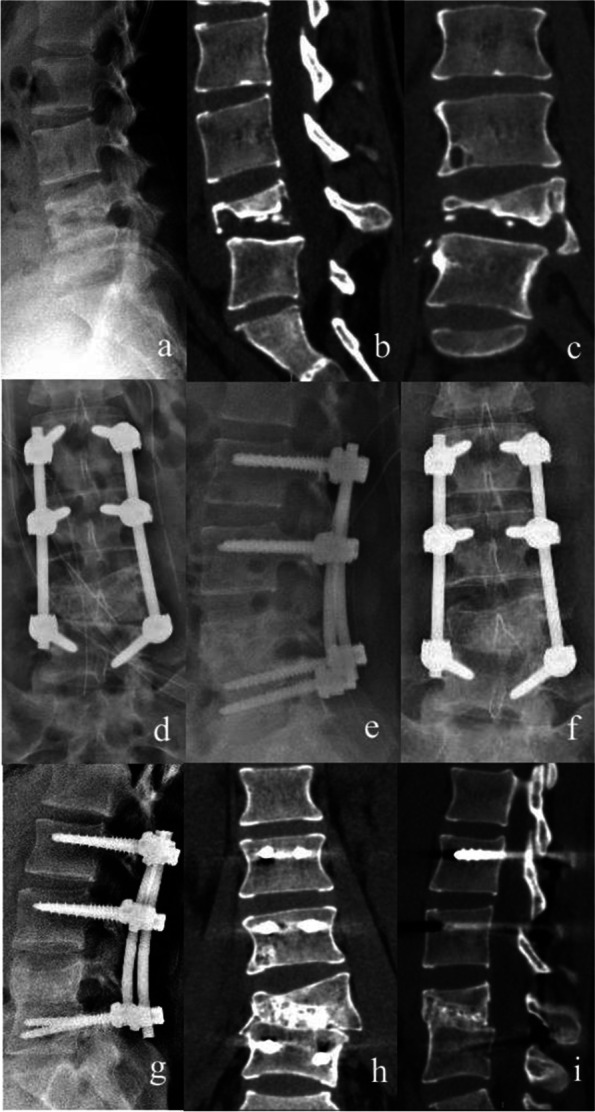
A 22 years old female diagnosed as L4-5 tuberculosis and treated with non-structural bone graft. a-c Preoperative X-ray and CT scan. d-e Postoperative X-ray. f-i X-ray and CT scan at last follow-up
